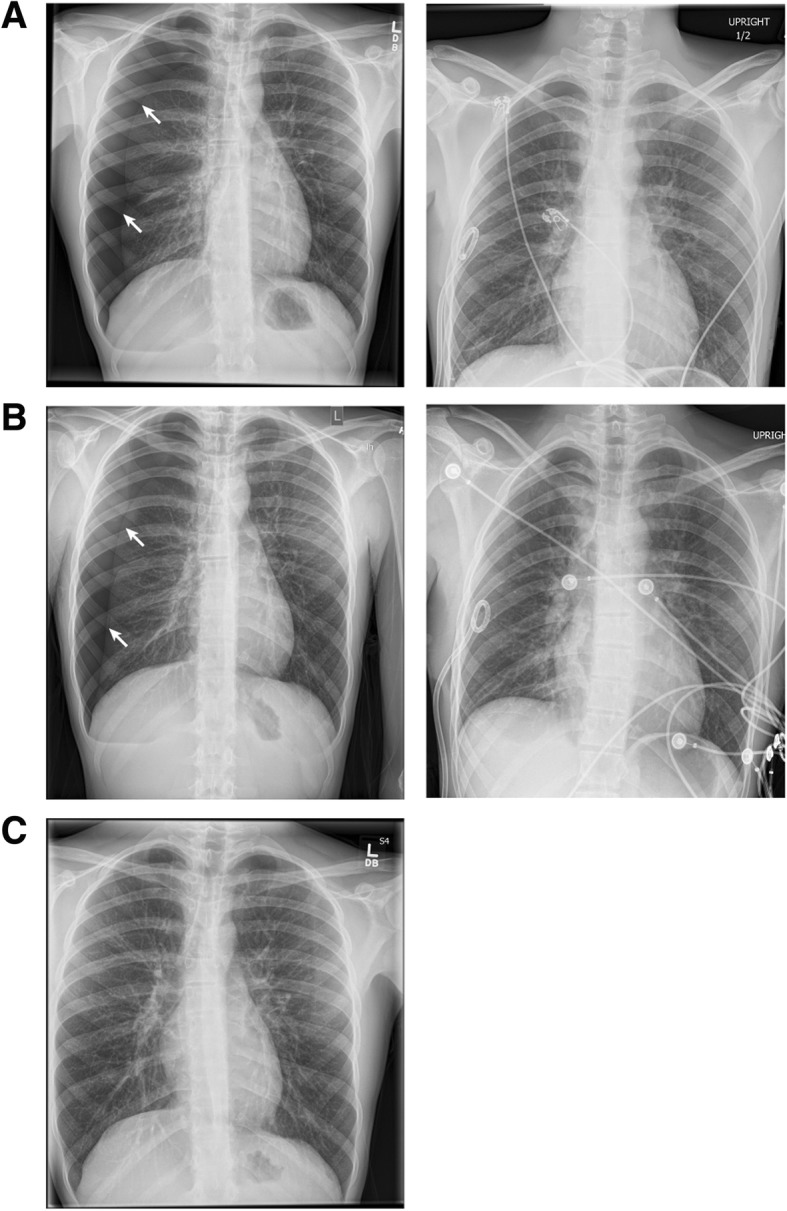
Chest X-ray at initial presentation demonstrating a right-sided pneumothorax (*arrow*) and resolving right-sided pneumothorax after pigtail chest tube placement for initial (a) and recurrent (b) spontaneous pneumothorax. (c) Sustained recovery from recurrent spontaneous pneumothorax two weeks after the removal of chest tubes
